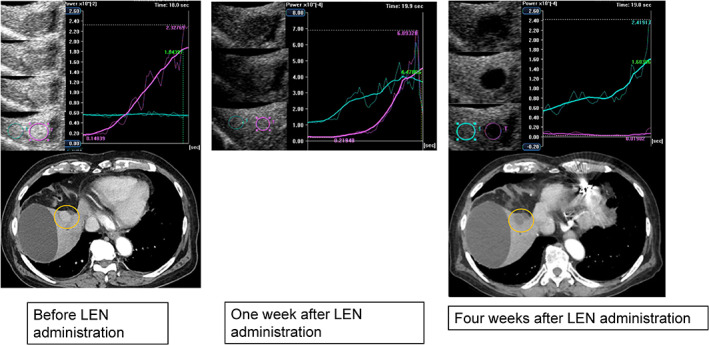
Clinical example of a good responder. An 87‐year‐old man with a history of hepatitis non‐B, non‐C virus cirrhosis underwent central hepatic bisection resection, and transcatheter arterial chemoembolization (TACE) for advanced hepatocellular carcinoma. Subsequently, he underwent radiofrequency ablation (RFA) under laparotomy for HCC recurrence. After RFA under laparotomy, the biliary cyst was complicated. HCC recurrence was observed in S5. TACE was difficult because of the presence of bile cysts. Radiation therapy was difficult near the intestine, and LEN administration (8 mg/day) was initiated. Gray‐scale ultrasonography showed a mosaic‐pattern tumor, of diameter 23 mm, in S5. This tumor was established as the target lesion. Images were taken with blood vessels and cysts near the tumor as landmarks. The left image was taken before LEN administration, the center was taken 1 week after LEN administration, and the right image was taken and TCA was performed 4 weeks after LEN administration. In each period, the CEUS, TCA, and CT were used for evaluation. TCA could measure the brightness of perflubutane. The brightness unit was automatically adjusted according to the numerical values of the HCC and background. Before administration, the peak intensity of the tumor was higher than that of the background liver. One week after LEN administration, the TCA results of the tumor and background liver were similar. Four weeks after LEN administration, it was reversed and the TCA result of the background liver was higher than that of the tumor. Four weeks after LEN treatment, a dynamic CT scan in the arterial phase showed a hypovascular lesion in S5, which was reduced. This therapeutic response was described as a partial response
